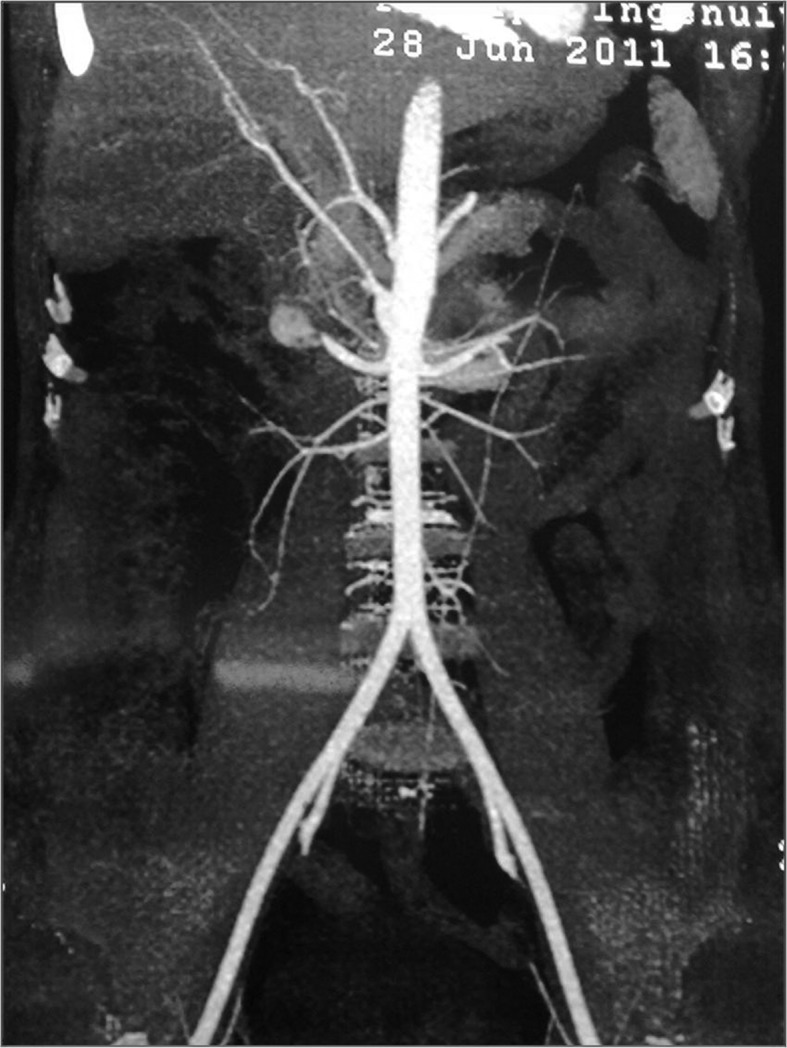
Contrast enhanced CT revealed a solitary insulinoma in the head of pancreas.
